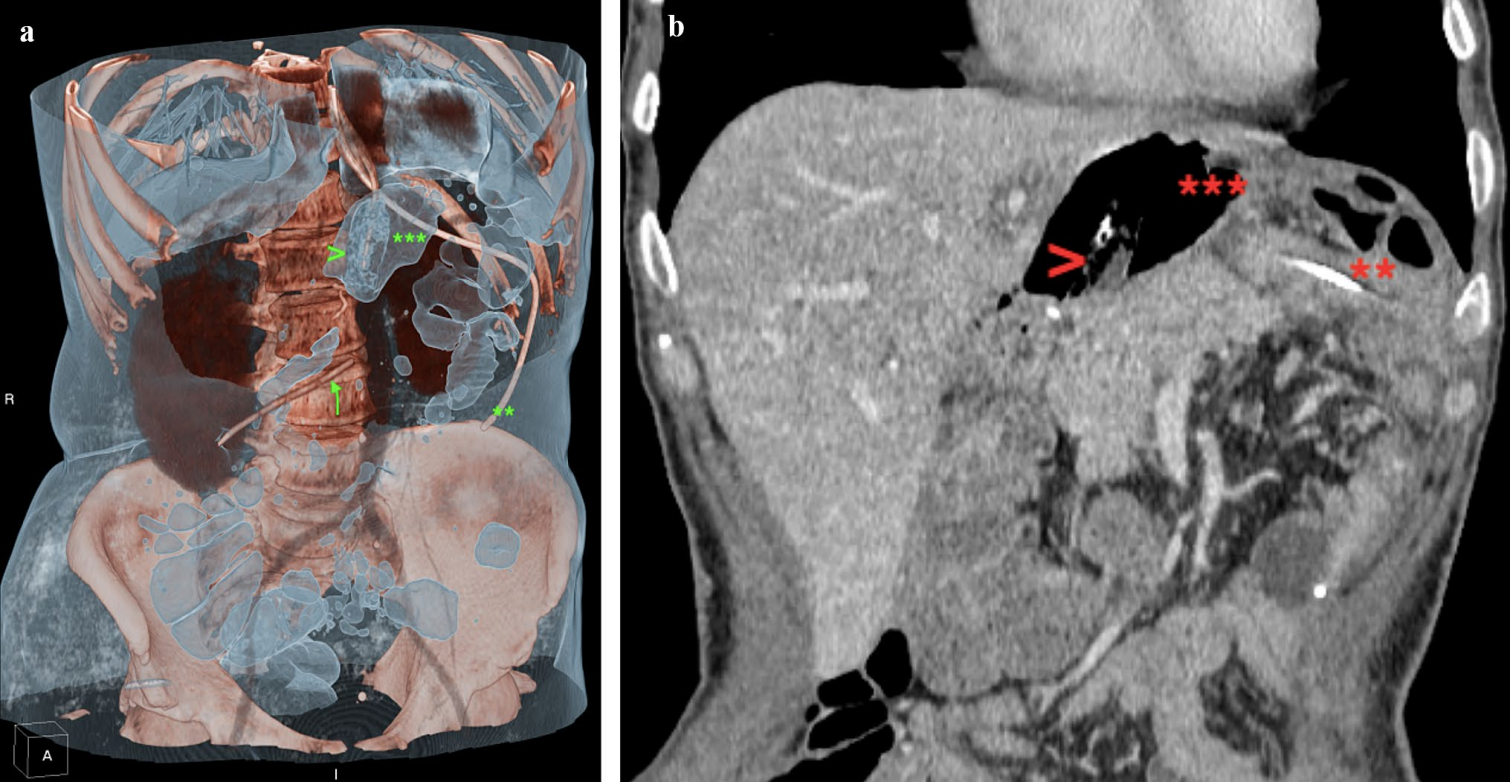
3D CT reconstruction (Fig. 1a) and CT scan (Fig. 1b) of esophagojejunal anastomotic leak (patient *n* 2) with esosponge in place. > Sponge; nasojejunal feeding tube; *wound cavity; arrow: abdominal drain
